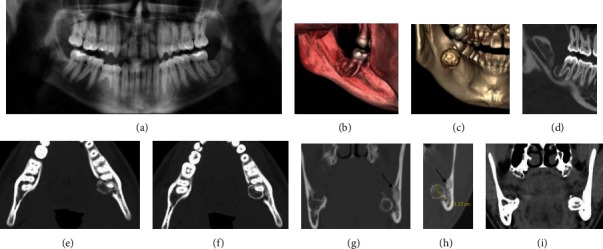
(a) Case #2 panoramic radiograph. (b and c) 3D CT reconstruction, viewed from the lingual aspect. (d) CBCT sagittal view. (e–f) Bone window CT axial views. (g–h) Bone window CT coronal views. (i) Soft tissue window CT coronal view. Panoramic radiograph was taken 8 months postsurgical extraction of the impacted tooth #17. An exophytic mixed density lesion, measuring 1.2 × 1.0 × 1.1 cm, emanated from the distal root of tooth #18. The border of the lesion is well-demarcated with a sclerotic rim surrounded by a lucent rim. Small area of bony sclerosis is noted at buccal and superior of the lesion (arrows) (g and h). There was no evidence of root resorption at associated root of #18. Unlike case 1, there is no evidence of expansion, new bone formation, or adjacent soft tissue edema (i).
